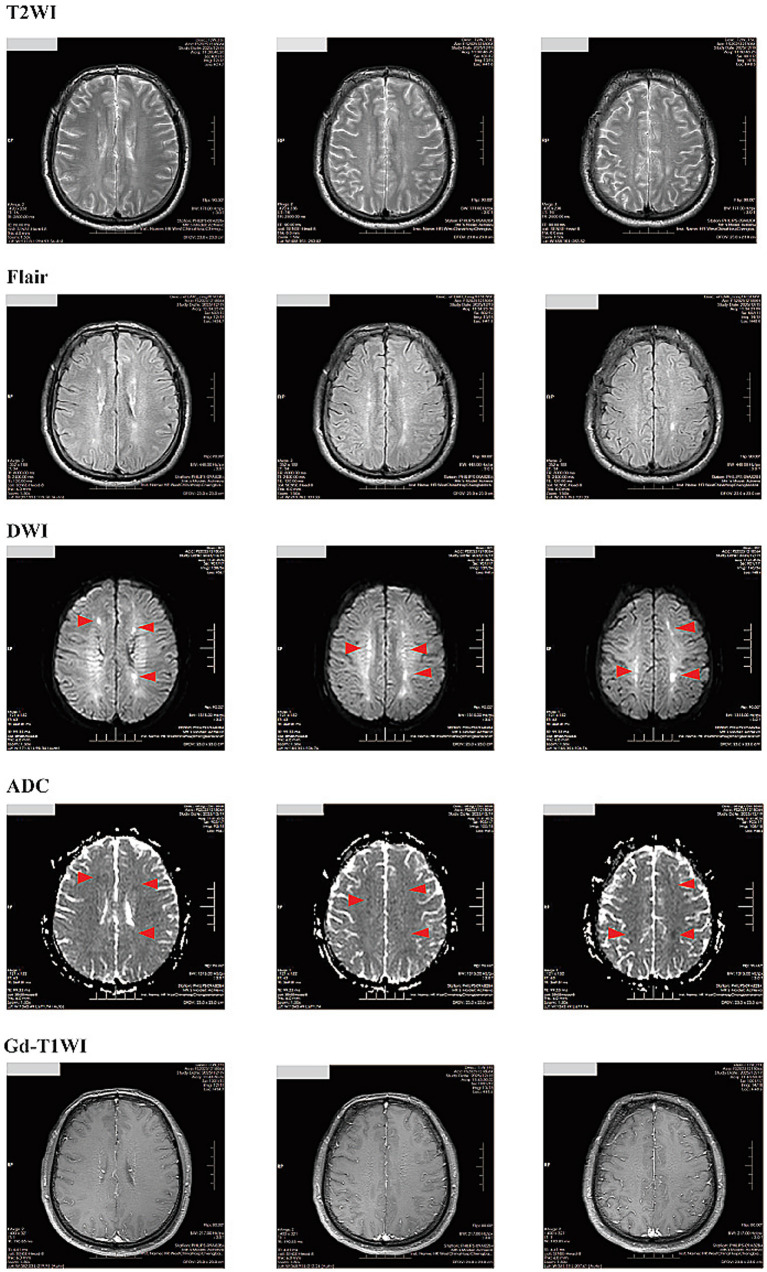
Cranial neuroimaging findings. This figure presents the patient’s cranial magnetic resonance imaging (MRI) scans, including T2-weighted imaging (T2WI), Fluid-Attenuated Inversion Recovery (FLAIR), Diffusion-Weighted Imaging (DWI), Apparent Diffusion Coefficient (ADC), and Gadolinium-enhanced T1-weighted imaging (Gd-T1WI). Multiple punctate and small patchy lesions exhibiting restricted diffusion are evident within the bilateral frontoparietal lobes and centrum semiovale, as indicated by the red arrows. No contrast enhancement was observed on post-gadolinium sequences.
